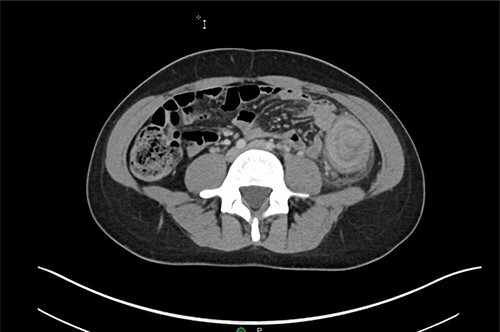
Axial CT demonstrating segmental wall thickening of the descending colon with adjacent inflammation favoring acute diverticulitis or segmental colitis.
